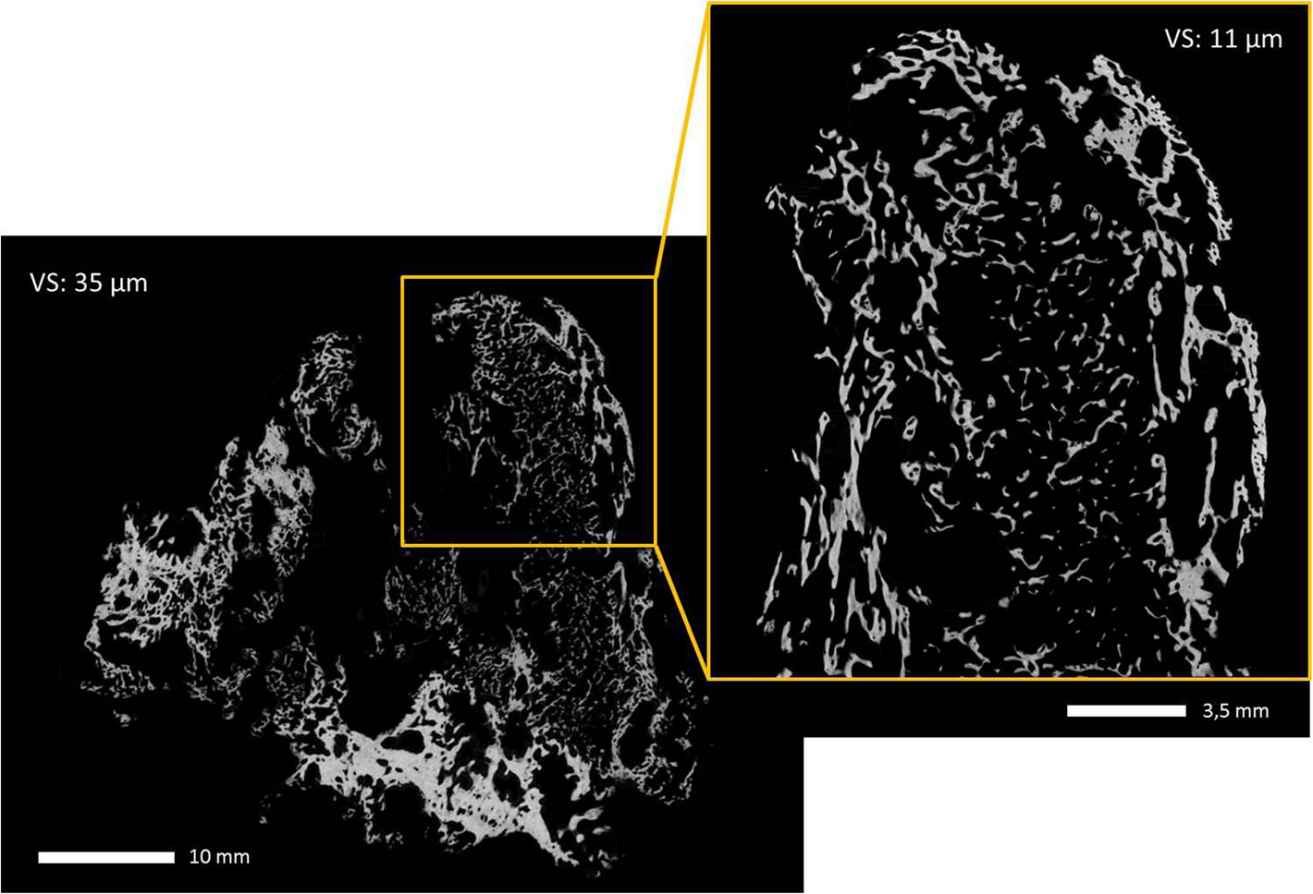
Microcomputed tomography image of one sample
